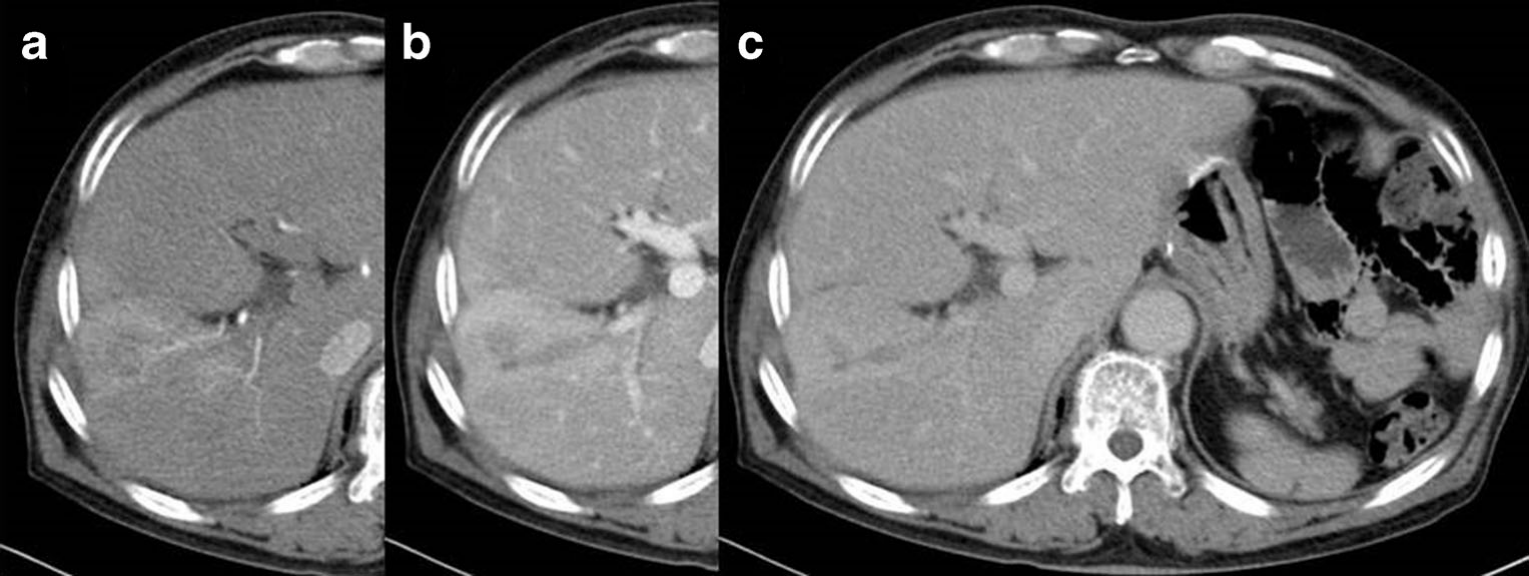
Abdominal contrast CT images of the liver tumor at the initial visit to our hospital in August 2009. a Arterial phase. b Portal phase. c Equilibrium phase
